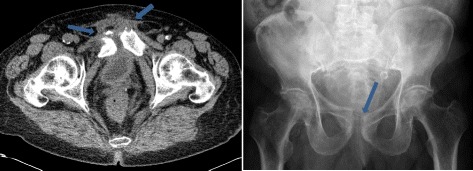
Symphysitis (computed tomography scan, *left*) and dilatation of the symphysis fissure and osseous erosions (conventional radiography, *right*) (*arrows*)
